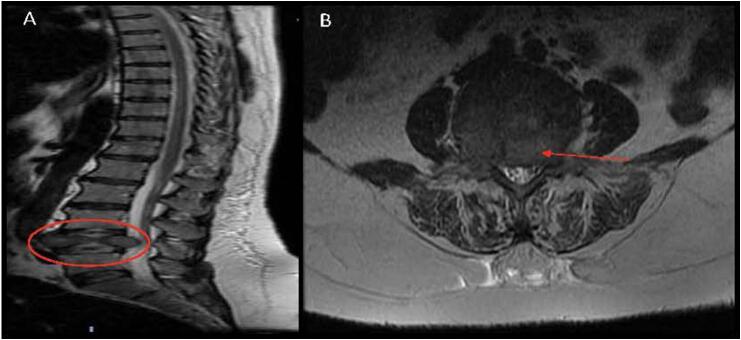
Patient 3: Preoperative MRI. (A) Sagittal T2-weighted MRI showing vertebral body collapse, retropulsion, and stenosis at the L4 level. (B) Axial T2-weighted image showing stenosis and retropulsion at the same level.
